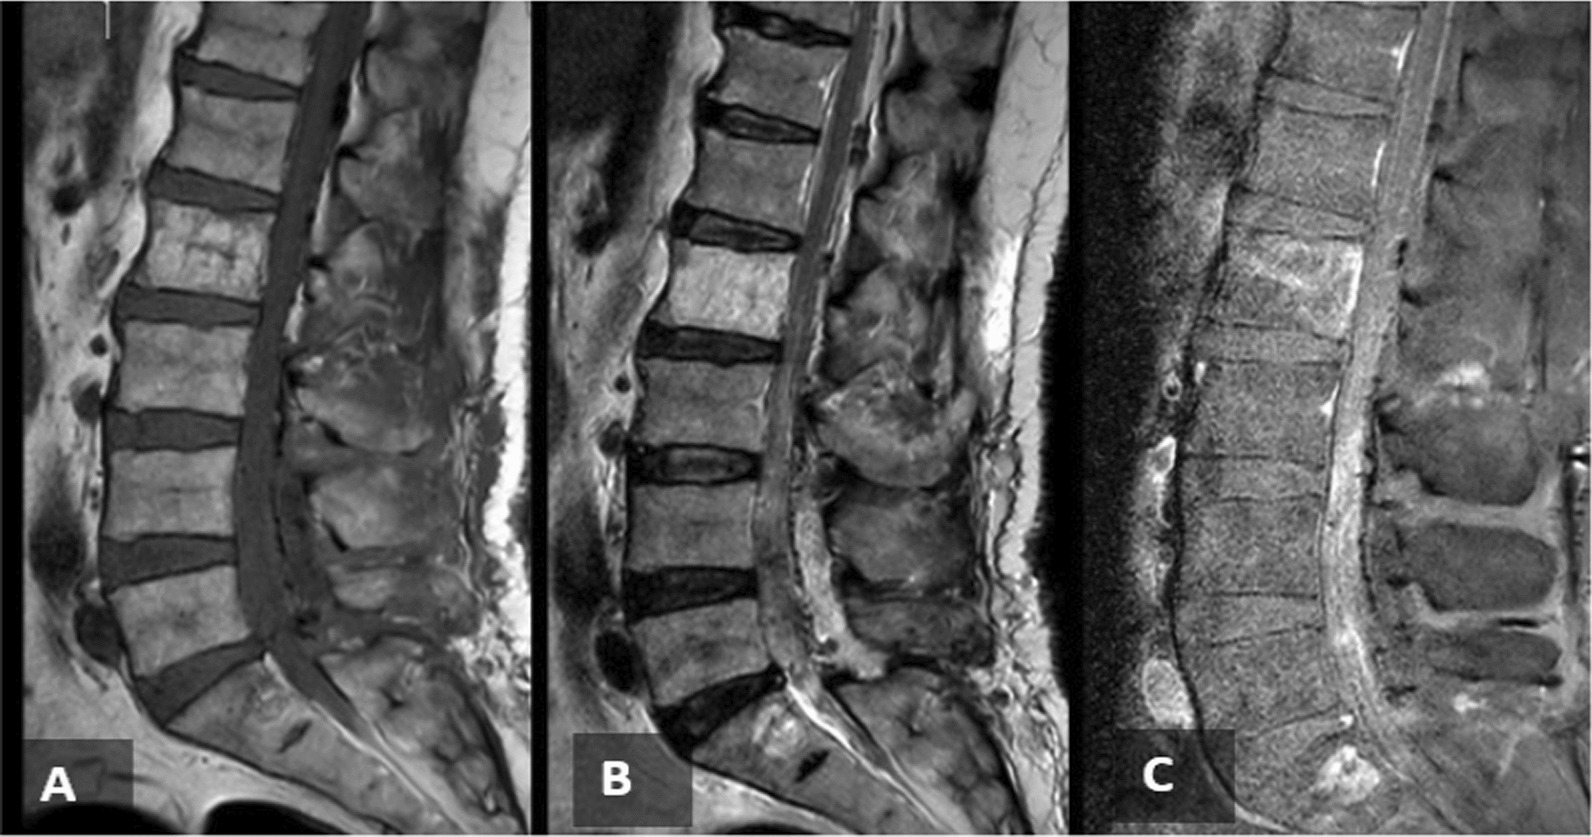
A, B and C, sagittal T1, T2, and T1 contrasted postoperative MRI imaging of the lumber spine respectively, showing gross total resection of the lesion with postoperative changes
